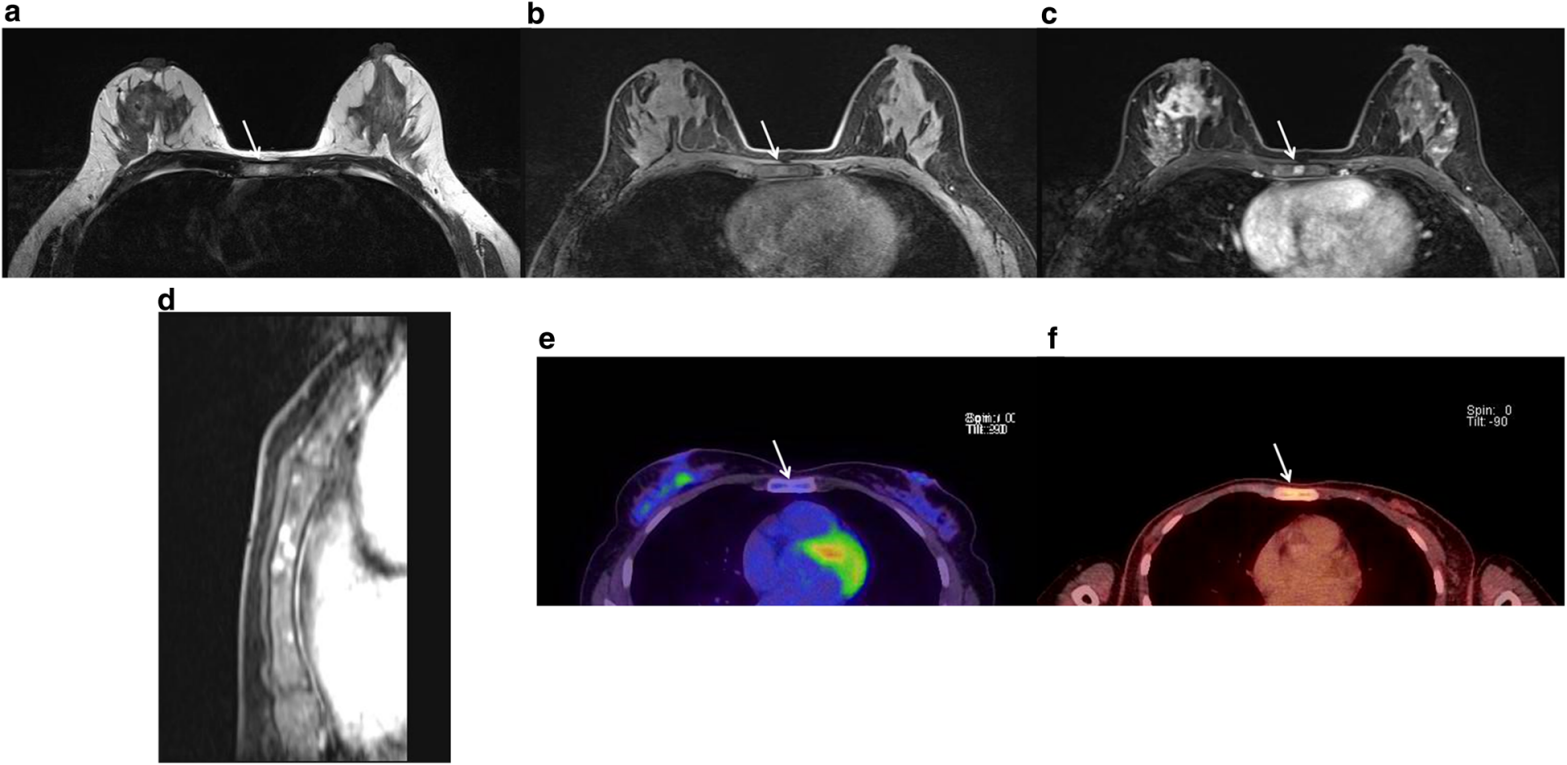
Early detection of bone metastases with MRI. Case of a 43-year-old female patient diagnosed with stage I breast cancer. Multiple sternum lesions (*arrow*) shown as hyperintense nodules on T1WI (a), T2WI (b), with positive contrast enhancement (c), and multiplicity (d) on contrast-enhanced T1WI. On initial PET/CT (e), no definite uptake was noted, but 7 months later, FDG uptake was seen on follow-up PET/CT (f)
